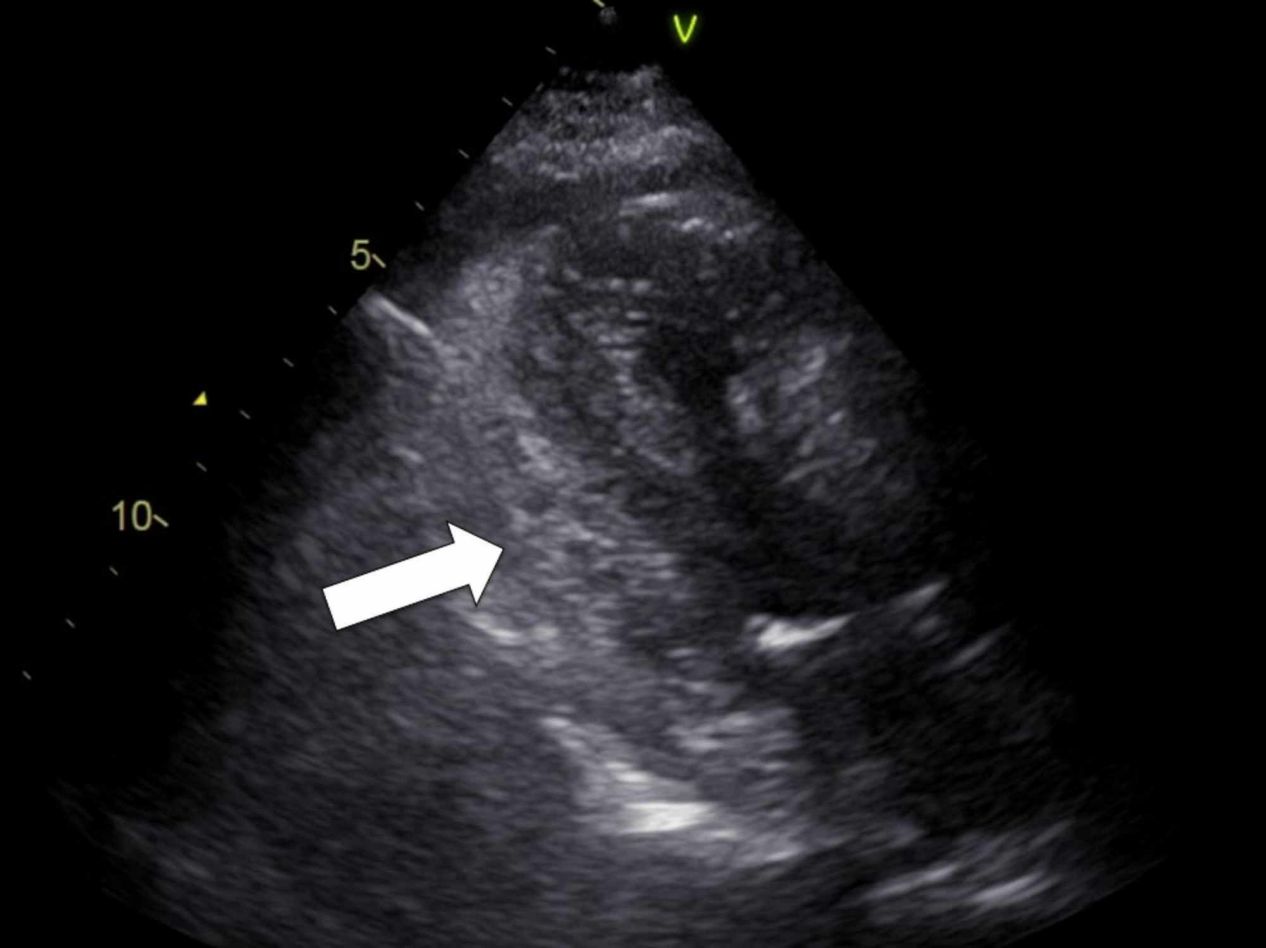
Ultrasound on arrival The echocardiographic findings suggest cardiac tamponade by hematoma (arrow: hyperechoic area). The heart was compressed by hematoma.
